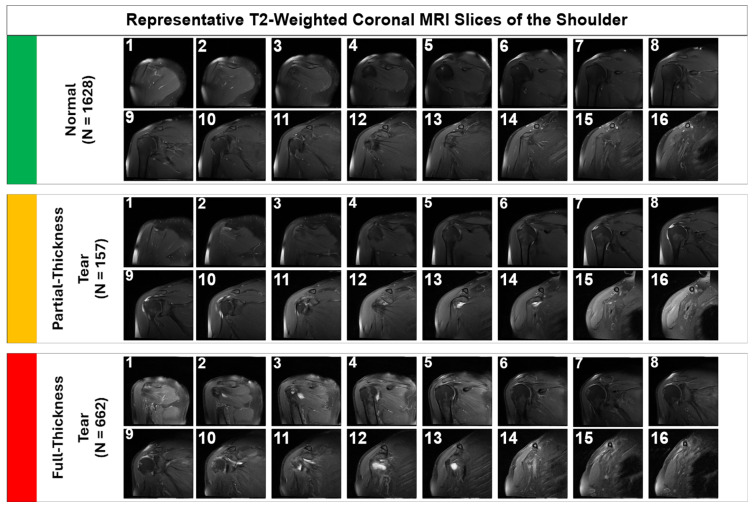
Representative T2-weighted coronal MRI slices from the dataset. (Top Row) Normal tendons showing uniform low signal intensity. (Middle Row) Partial-thickness tears characterized by focal hyperintensity on the articular side of the tendon. (Bottom Row) Full-thickness tears demonstrating complete tendon discontinuity and significant fluid accumulation.
